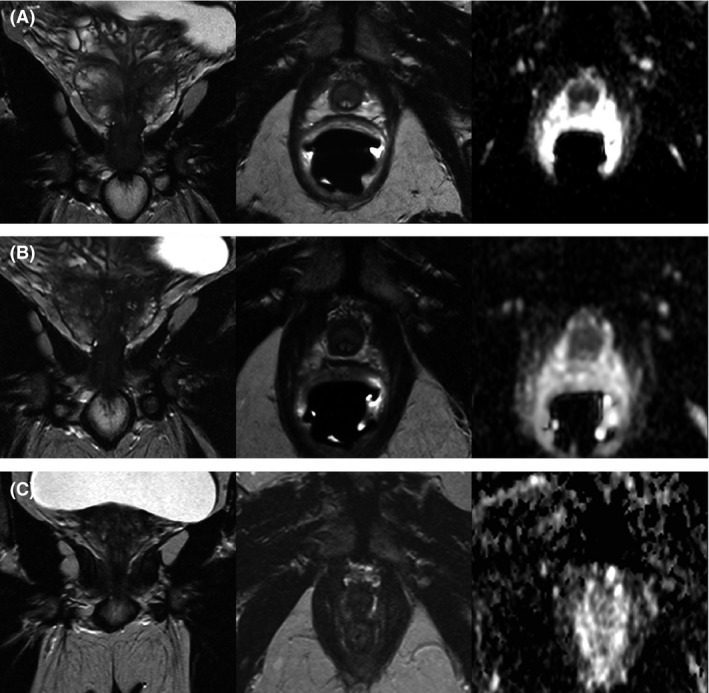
Preinterventional magnetic resonance images, showing t2 coronal (left), t2 axial (middle), and ADC‐map images (right) to calculate ADC values of the tumor site. MRI images in A and B were acquired with an endorectal coil to increase sensitivity. (A) Pre‐ADT therapy, ADC (measured in central lesion) approx. 568 ± 89 mm^2^/sec. (B) 4 weeks into AHD therapy, ADC approx. 739 ± 36 mm^2^/sec. (C) After three months of ADT and 1 day before intervention, ADC approx. 700 ± 145 mm^2^/sec. Images show the tumor growth along the urethra. Initial prostate volume was approx. 35 mL, but got reduced to 19 mL pretreatment. A detailed description is given in the text.
